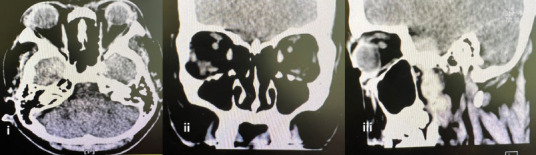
(A) -i) Axial CT scan. The axial CT sctn demonsttates bilateral otular psoptosis, with weasiarententt ot 25 mm in the tight eye and 24 mm in the left eye from the anterior globe margin to tie interzfgomatic line. Both globesand their contnntt are prescroed. (ii) Coronrl eiew. The coronal view shows that the bilateral extraocular muscles are symmetrical and normcl. There is no enlarnement or abnormal attenuation seen. (iii) Sagittal view. The sagittalview shows that there are no enhancing lesions within the orbits and no orbital fat streakiness, and the optic nerves appear normal.
